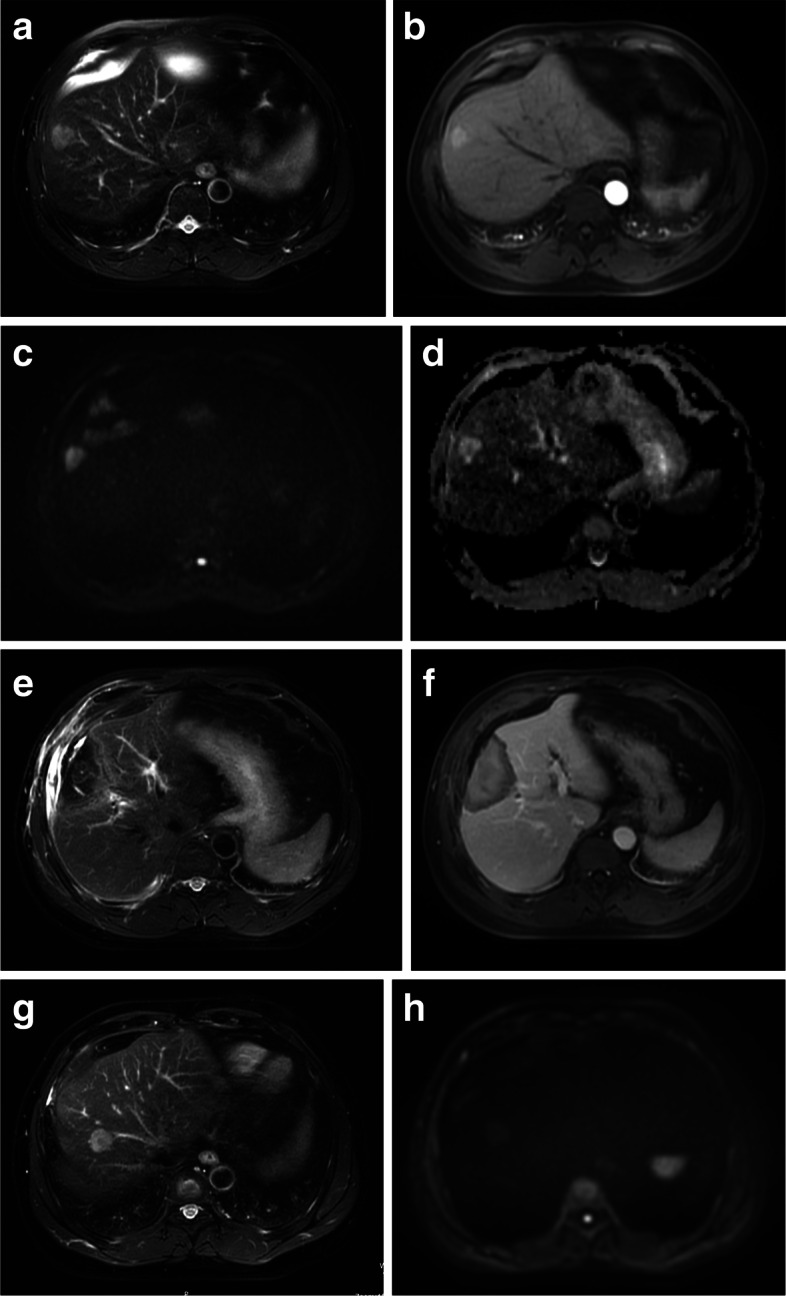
A 55-year-old man with a 2.5-cm single hepatocellular carcinoma (HCC). A Axial breath-triggered single-shot T2-weighted magnetic resonance imaging (MRI) showed a hyperenhancing mass in the S8 segment of the right liver near the hepatic margin. B Significant enhancement of the mass was seen in the arterial phase, with an ill-defined margin at the mass. C Axial single-shot diffusion-weighted imaging (DWI) (b = 800 s/mm^2^) and D apparent diffusion coefficient (ADC) maps showing a visually assessed diffusion restriction of the tumor. ADC and eADC were 0.973 × 10^−3^ mm^2^/s and 0.215, respectively, and the recurrence score exceeded the optimal cutoffs, thereby indicating a high risk of recurrence. Although a sufficient ablative margin was obtained (MAM > 5 mm), meaning that complete ablation was confirmed (E–F), tumor recurrence occurred in the right liver at 18 months after complete ablation (G)
